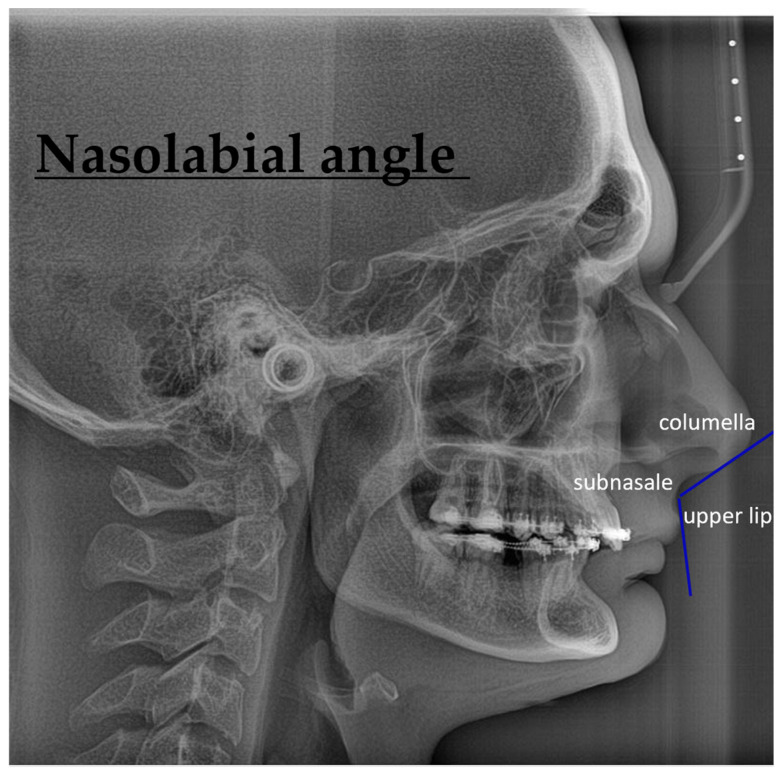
Lateral cephalometric radiograph showing the nasolabial angle, formed by the columella, subnasale, and upper lip.
